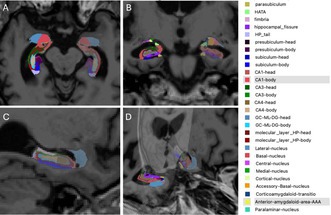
FIGURE 1 Representative segmentation of hippocampal and amygdala subfields on high‐resolution T1‐weighted MRI. (A) Axial, (B) coronal, (C) sagittal, and (D) three‐dimensional views with color‐coded subregions.
